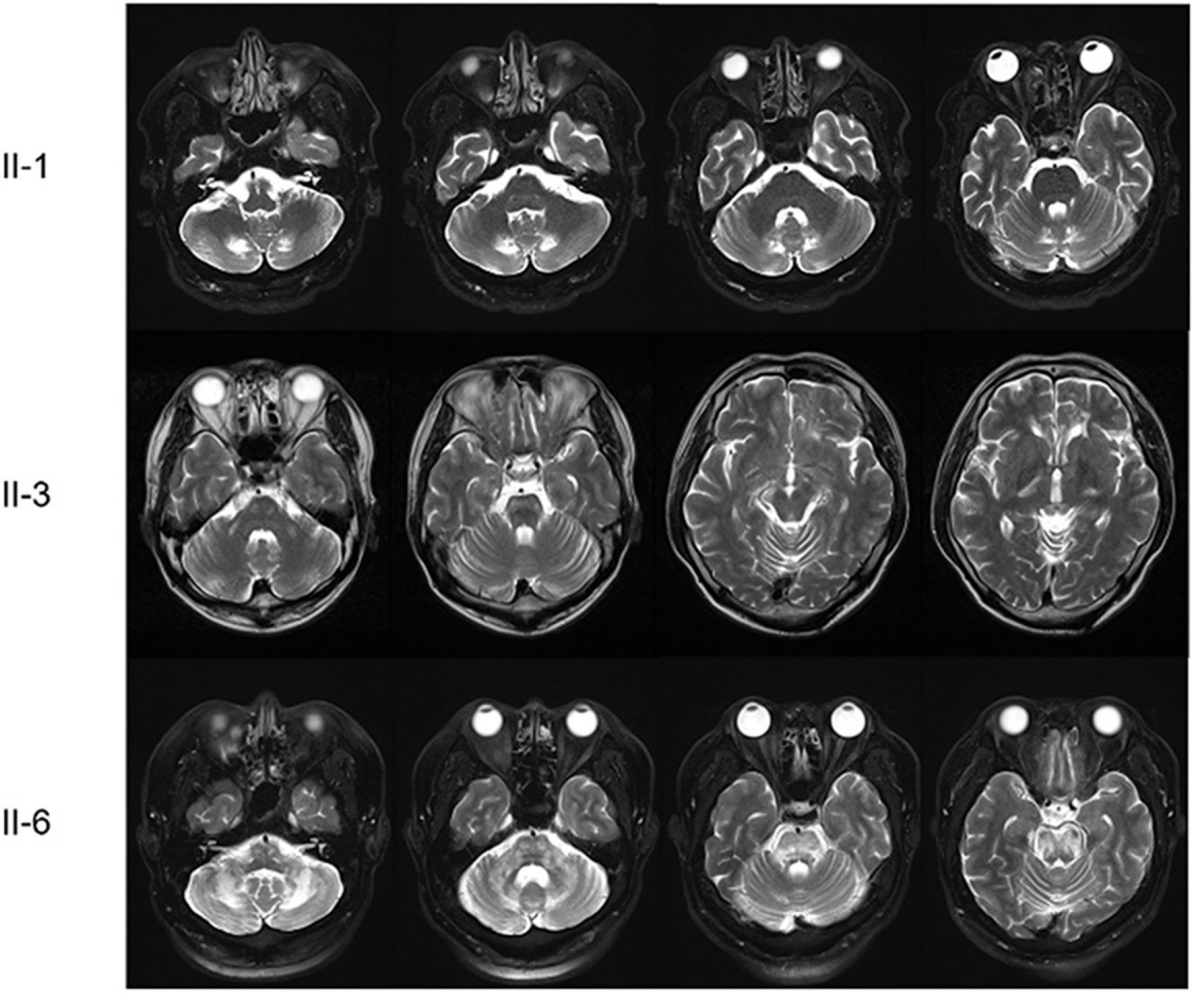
Brain MRI scans of the patients. The proband (II-1) had symmetric T2-weighted hyperintensities in the dentate nuclei and the surrounding cerebellar white matter. The first younger brother (II-3) presented brainstem and cerebellar atrophy with asymmetric T2-weighted hyperintensities in the middle cerebellar peduncles, cerebral peduncles, posterior limbs of the internal capsules, and periventricular white matter of the frontal lobe. The third younger brother (II-6) had diffuse T2-weighted hyperintensities in the midbrain, pons, middle cerebellar peduncle and cerebellum.
